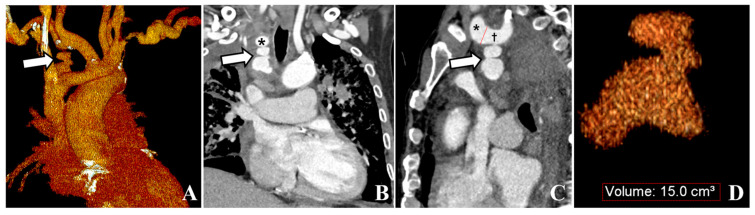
A 77-year-old man shows an irregular-shaped pseudoaneurysm at the most proximal portion of the subclavian artery, extending to the mediastinum. (A) The 3D volume rendering image shows a pseudoaneurysm (white arrow). (B) The coronal reformatted contrast-enhanced CT demonstrates the pseudoaneurysm (white arrow) extending to the mediastinum. Note the subclavian artery (black asterisk). (C) The sagittal reformatted contrast-enhanced CT demonstrates the pseudoaneurysm (white arrow) arising from the subclavian artery (black dagger). Note the common carotid artery (black asterisk). (D) The 3D volume rendering image shows an irregular-shaped pseudoaneurysm. The volume of pseudoaneurysm is approximately 15 cc.
